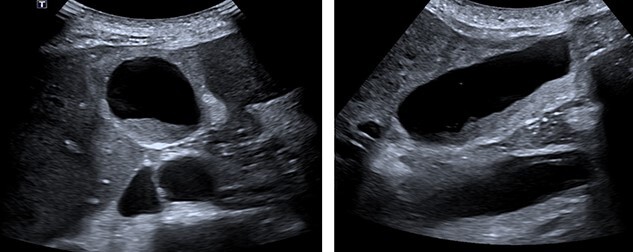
Abdominal ultrasound. A layer of mobile sludge and thickened gallbladder walls. Several liver lesions with mixed echogenicity were noted, several adjacent to the gallbladder fossa.
